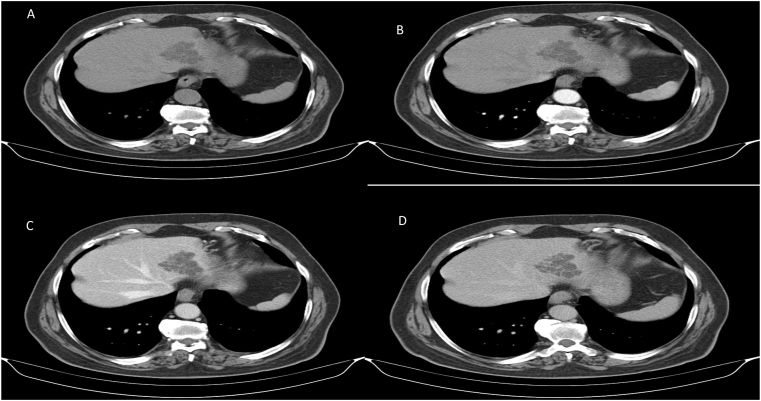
Image 1a: CT axial without contrast: a lobulated hypointense, cystic-type mass is depicted in liver segment ΙΙ, with 5 × 4cm dimensions. 1b: CT axial arterial phase shows the multilocular cystic mass with a few mild enhancing internal septa.1c: CT axial portal venous phase shows the well-defined multilocular intrahepatic cystic mass with multiple enhancing internal septa. There is no dilatation of the intrahepatic biliary ducts.1d: CT axial delayed phase shows the thick internal septa with persisted enhancement.
